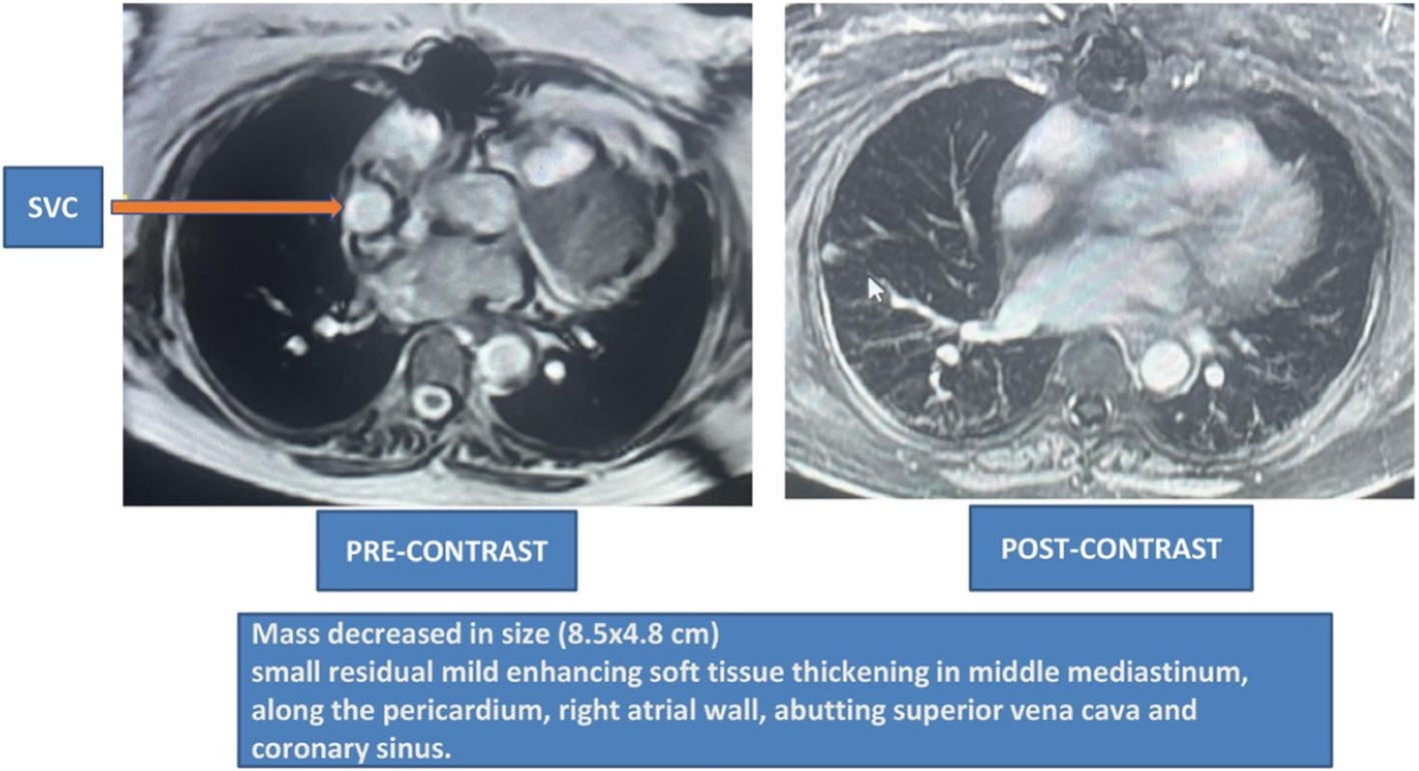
Cardiac magnetic resonance imaging showing a residual mass in the middle mediastinum with no avidity. SVC, superior vena cava
